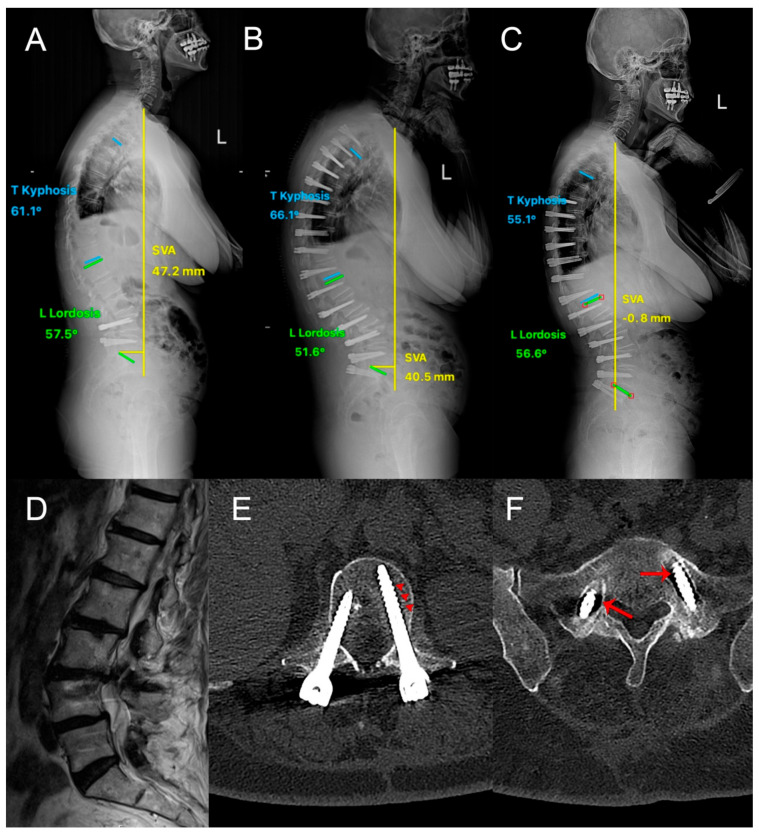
A 69-year-old female patient with osteoporosis (T: −2.8) who has been previously operated for lumbar spinal stenosis at L4–L5 presented with neurological claudication and sensory loss in both extremities. (A) Preoperative lateral standing X-ray showing sagittal imbalance. (D) Preoperative sagittal T2W MRI showing adjacent segment degeneration and spinal stenosis at L3–L4 and L2–L3. Two-stage dynamic stabilization with the Dynesys system was performed. Standing lateral X-ray (B) after 1st operation and (C) after 2nd operation, showing significant improvement in sagittal alignment. (E) Postoperative (2nd operation) axial CT scan showing successful osteointegration at L1 level, and (F) postoperative (2nd operation) axial CT scan showing marked screw loosening at S1 level. The areas of radiolucencies, as indicated by arrows, demonstrate screw loosening, while arrowheads highlight osteointegration.
